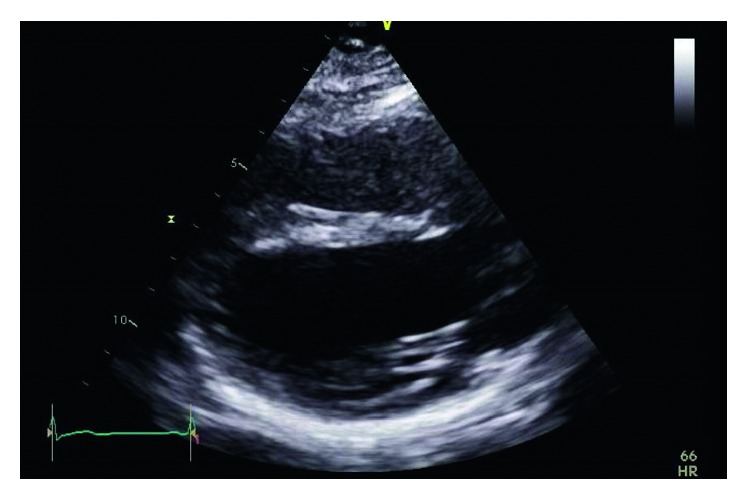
Dilated right ventricle on echo parasternal long view.
